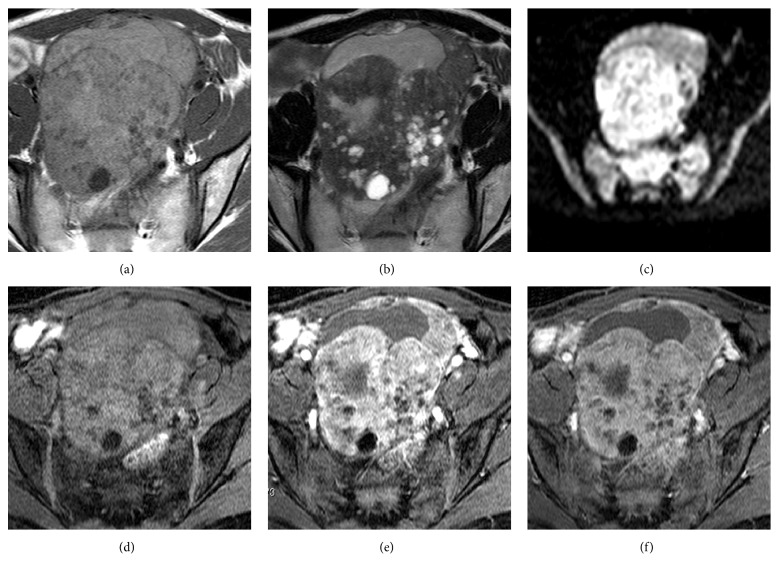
(a) T1-weighted magnetic resonance imaging depicting a mass in the right adnexal region with high signal intensity relative to that of the myometrium. (b) On T2-weighted imaging, the solid component of the mass exhibited slightly low signal intensity, and the large cystic component exhibited high signal intensity. (c) Diffusion-weighted imaging depicting a mass with high signal intensity relative to that of the endometrium. (d) On precontrast fat-saturated T1-weighted imaging, the mass exhibited slightly high signal intensity. (e) On early-phase contrast-enhanced fat-saturated T1-weighted imaging, the mass exhibited strong high signal intensity. (f) On delay-phase contrast-enhanced fat-saturated T1-weighted imaging, the mass exhibited slightly high signal intensity.
